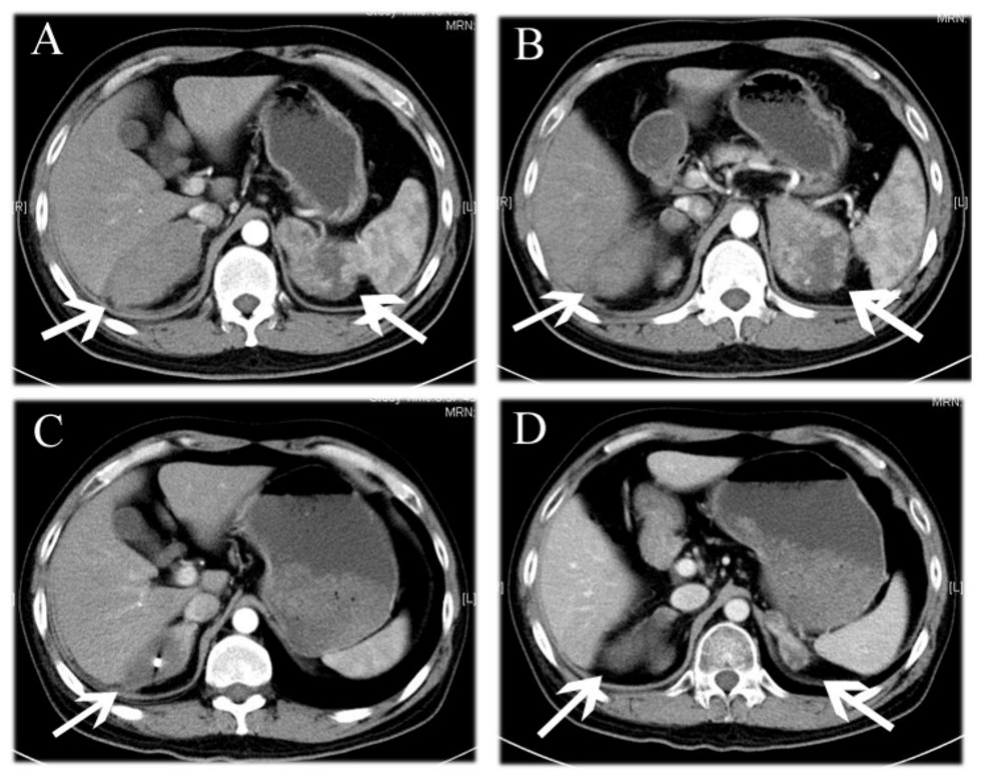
Comparison before CyberKnife treatment with that at 2 months after treatment. A 40 years old male patient with adenocarcinoma involving the right lung with metastases in the left and right adrenal after 4 cycles of chemotherapy. (A, B) Abdominal transverse enhanced CT scanning shows bilateral adrenal metastasis. (C, D) Abdominal transverse enhanced CT at 2 months after CK treatment shows bilateral metastases were significantly reduces with the previous. The patient had varying degrees of lower back and abdomen swelling pain before treatment, the symptom were relieved at the time when the patient was followed up.
